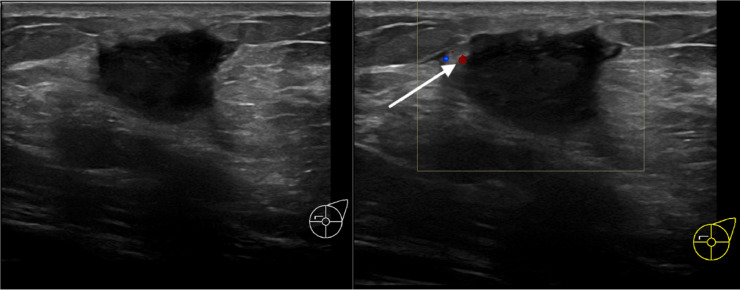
Ultrasound of the left breast with transversal orientation. Displays a hypoechoic mass in the in the upper quadrant at 10-o'clock with indistinct contours and posterior shadowing. Notably, there is a few color Doppler vascularization (white arrow).
